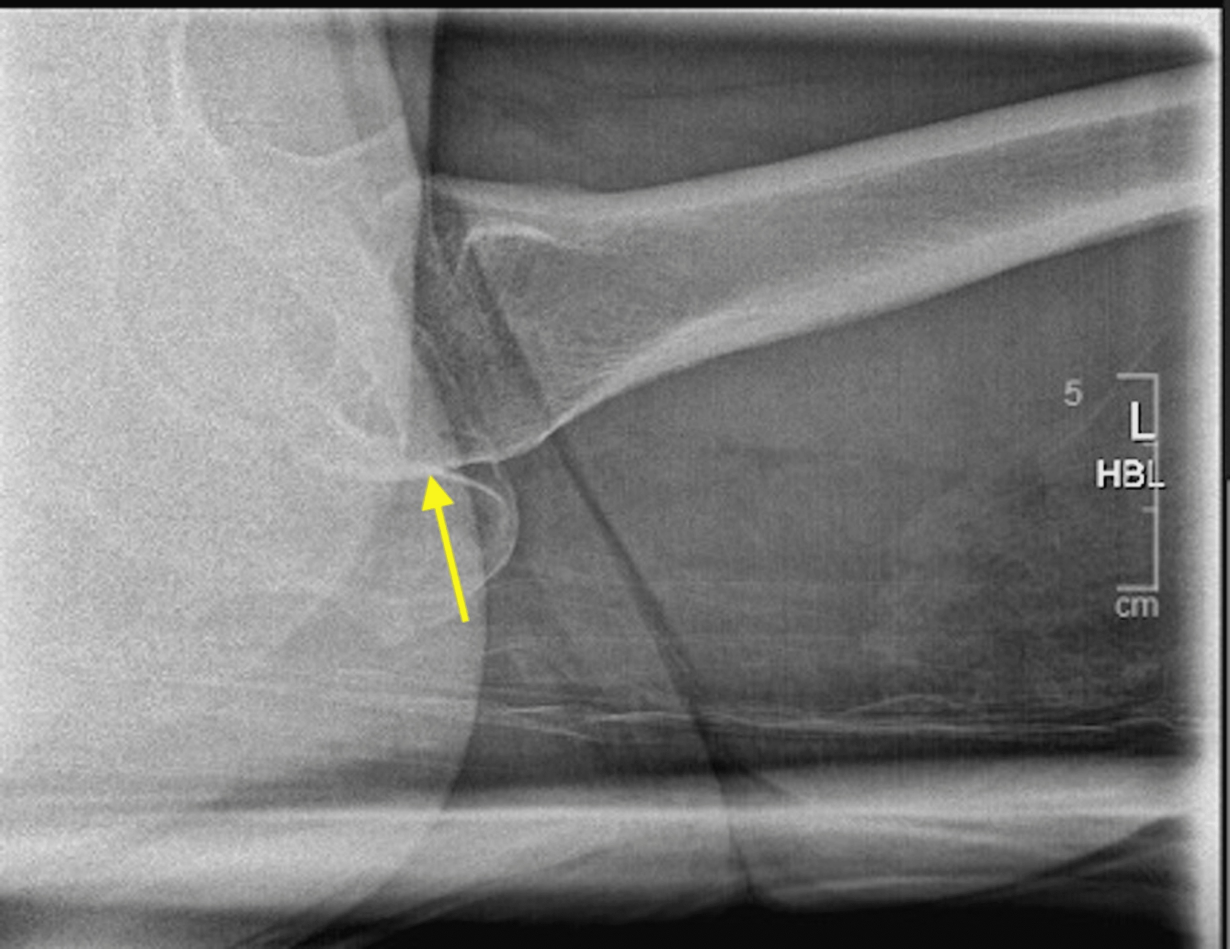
Pre-operative lateral X-ray
